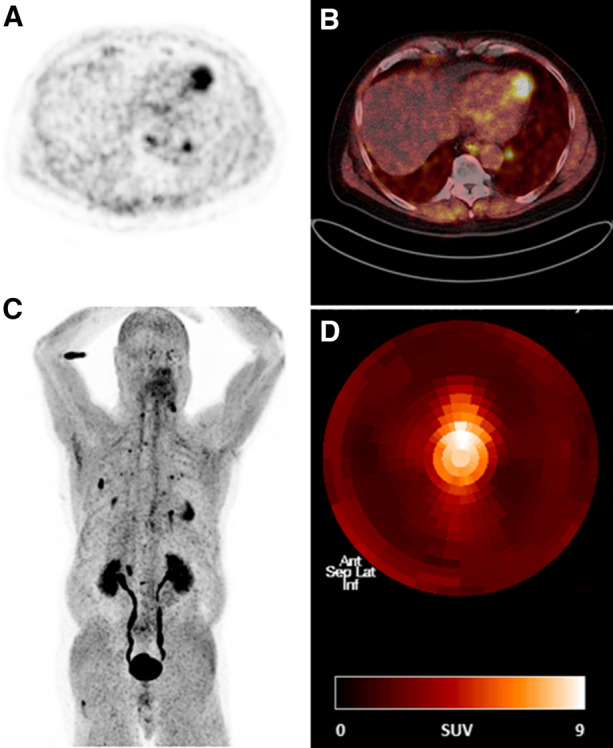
Localized apical FAPI uptake in a patient (patient #29) with papillary thyroid cancer. The whole-body FAPI scan reveals FAPI uptake in cervical lymph nodes as well as suspicion of pulmonary metastases. (A) Trans-axial slice of Ga-68 FAPI PET. (B) Fusion of trans-axial Ga-68 FAPI PET and low-dose CT. (C) Maximum intensity projection of the whole body PET. (D) Polar map demonstrating spatial Ga-68 FAPI uptake in the left ventricular myocardium
